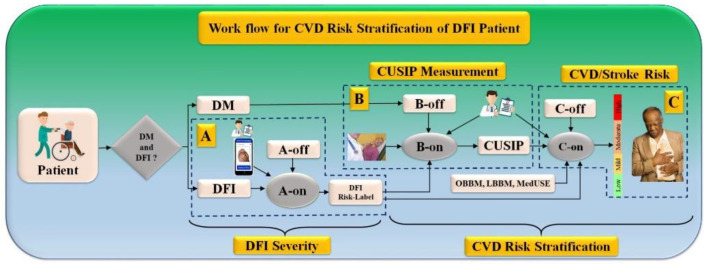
The overall architecture of CVD screening on DM and DFI patients. A-on: Online DL-based FDI severity system, A-off: Offline DL-based FDI severity system, B-on: Online DL-based Carotid wall quantification system, B-off: Offline DL-based Carotid wall quantification system, C-on: Online ML-based CVD Risk Assessment system, C-off: Offline ML-based CVD risk assessment system, DM: Diabetes Mellitus, DFI: Diabetic foot infection, CUISP: Carotid ultrasound image phenotype.
